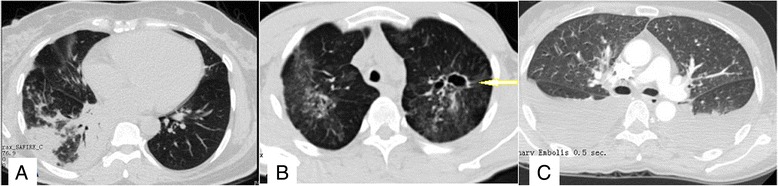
High-resolution computed tomography. High-resolution computed tomography shows interstitial infiltration and alveolar infiltration a, a cavitary lesion (arrow) b, and pleural effusion c
